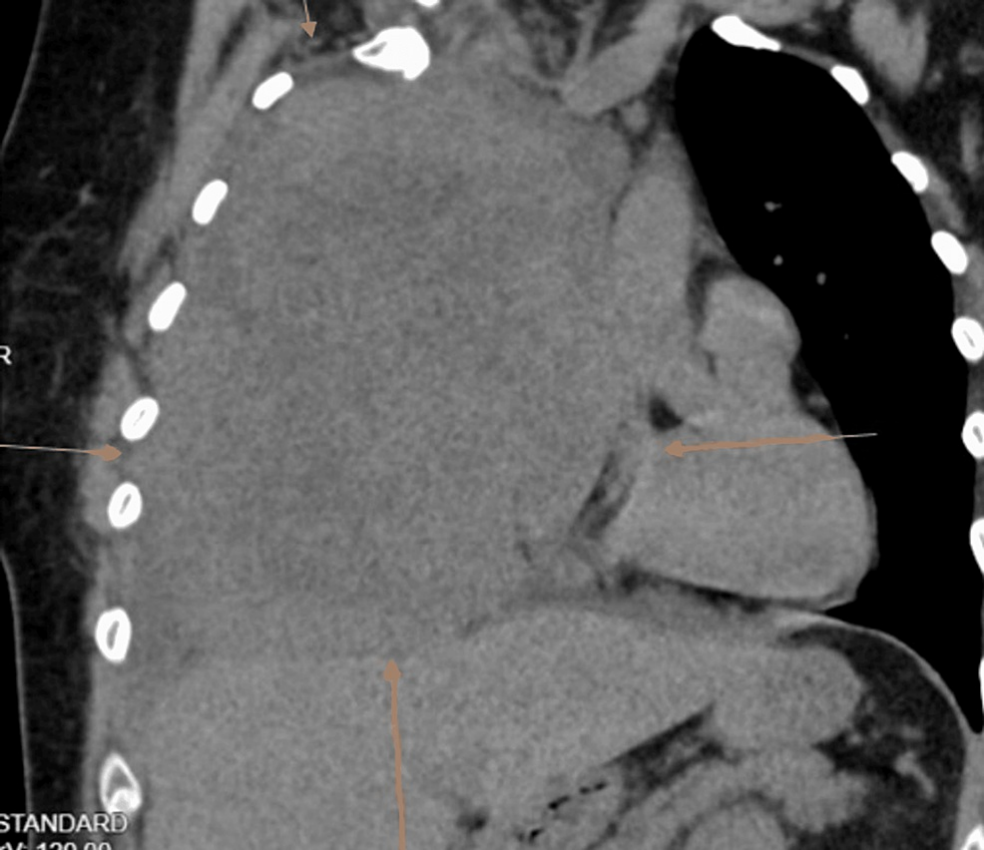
Computed tomography chest revealing right lung mass Arrows point toward the right lung mass
